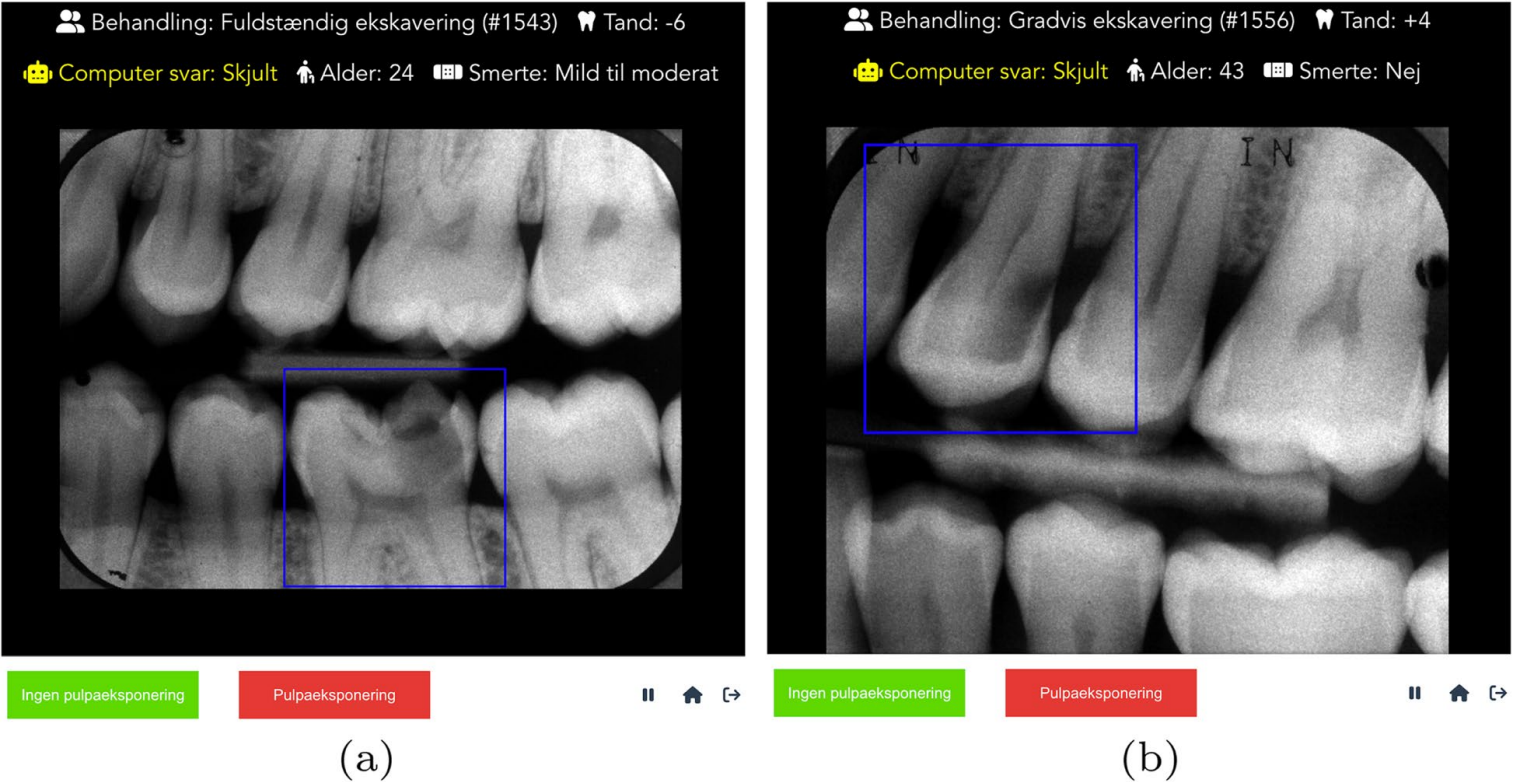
The experiment view of the platform designed to collect dentists’ predictions [19] Each sub-figure represents a different patient. All cases were extensive active stages (ICDAS 5, RC 5), with clinical details displayed at the top, including treatment type, tooth number, age, and pain information. The platform depicts all AI predictions as hidden during this experiment. A box highlights the affected tooth in each preoperative radiograph. The bottom bar includes two action buttons, representing “No pulp exposure” and “Pulp exposure” choices, respectively. It also features a progress bar indicating the participant’s current stage in the experiment. Patient (a) was treated with complete excavation and had pulp exposure. Patient (b) was treated with stepwise excavation and did not experience pulp exposure
